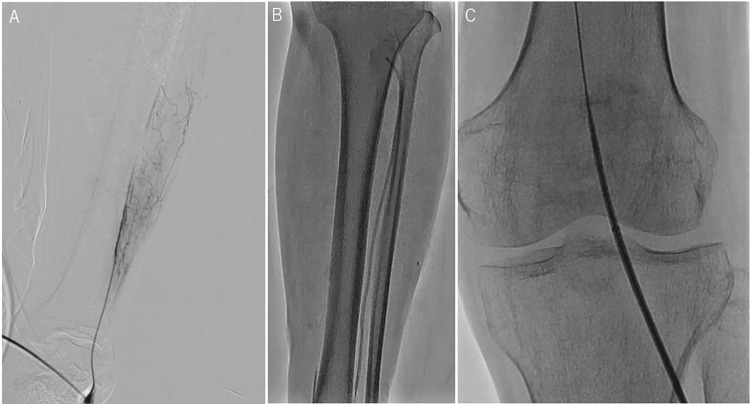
Steps of guide sheath insertion through the left dorsalis pedis artery (A) First, the left dorsalis pedis artery was punctured under duplex ultrasound guidance, and a 3-French sheath was inserted. (B) Second, a 0.014-inch guide wire was passed through the occluded lesion of the anterior tibial artery, which was reopened with standard balloon angioplasty using a 2.5- × 220-mm balloon. (C) Third, the 3-French sheath was replaced with a 5-French 53-cm sheath, and the guide sheath tip was placed at the middle part of the popliteal artery.
